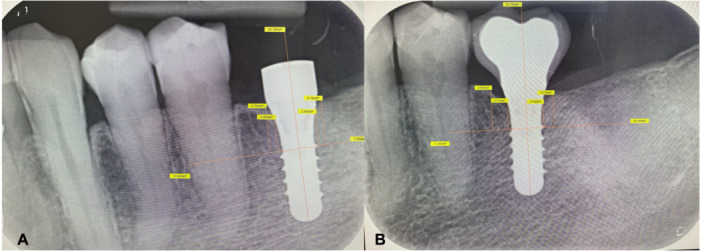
Radiographic bone level measurement for TL implants. A line was drawn along the central axis of the implant, and a perpendicular reference line was drawn touching the most coronal implant thread; the vertical distances from this reference line to the bone and to the mesial and distal bone peaks were then measured. (A) Measurement of the implant with the healing cap. (B) Measurement of the implant with the final crown.
